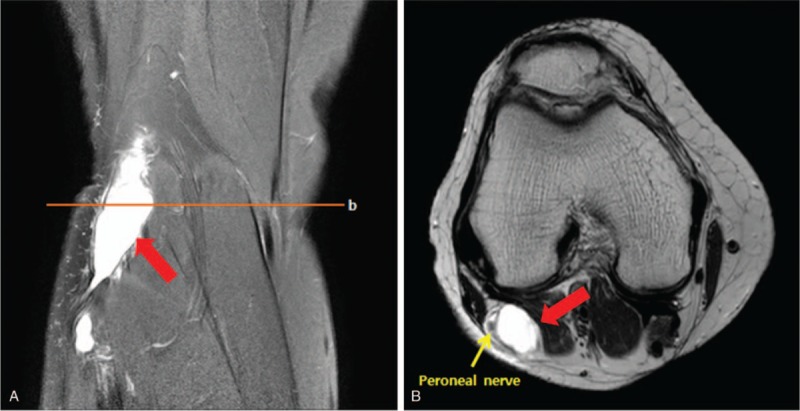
T2-weighted magnetic resonance images of the knee. (A) Coronal image of the popliteal fossa; (B) axial image at the distal femur level. The red arrows indicate the intraneural ganglion cyst in the common peroneal nerve. The yellow arrow indicates the compressed peroneal nerve.
